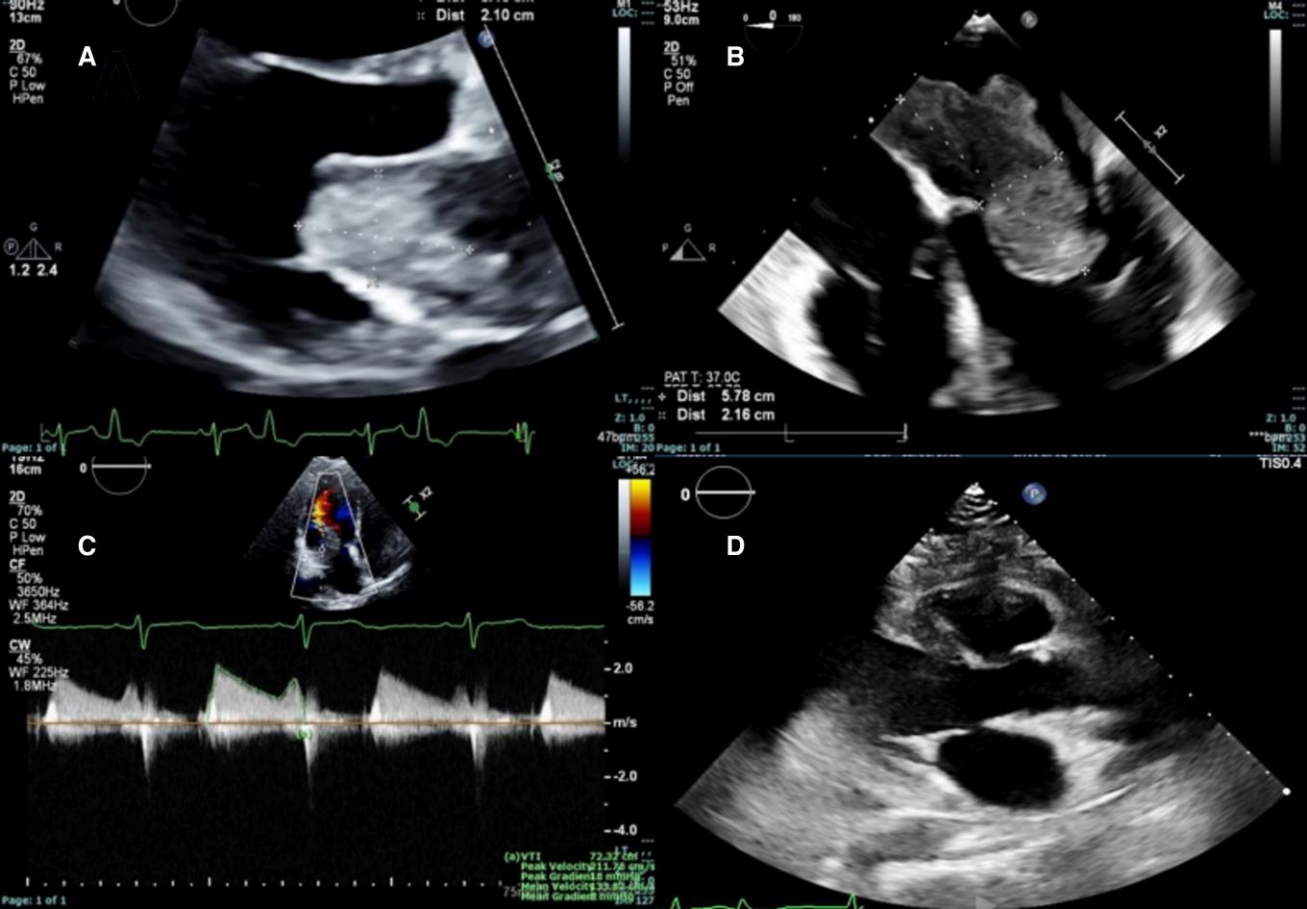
Echocardiogram findings of left atrial mass. *(A*) Parasternal long axis view of transthoracic echocardiogram showing a large polypoid mass in the left atrium obstructing left ventricular inflow during diastole. *(B*) Transoesophageal view of left atrial mass measuring 5.8 cm × 2.2 cm. *(C*) Mean pressure gradient across the mitral valve of 8 mmHg. *(D*) Post-operative echocardiogram in the parasternal long axis view demonstrating complete excision of the left atrial mass.
